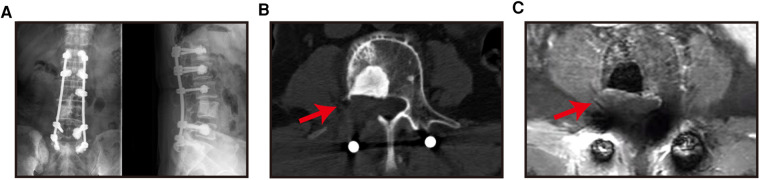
X-ray (A), CT images (B) and MRI images (C) of patients were reviewed 6 months after surgery.
